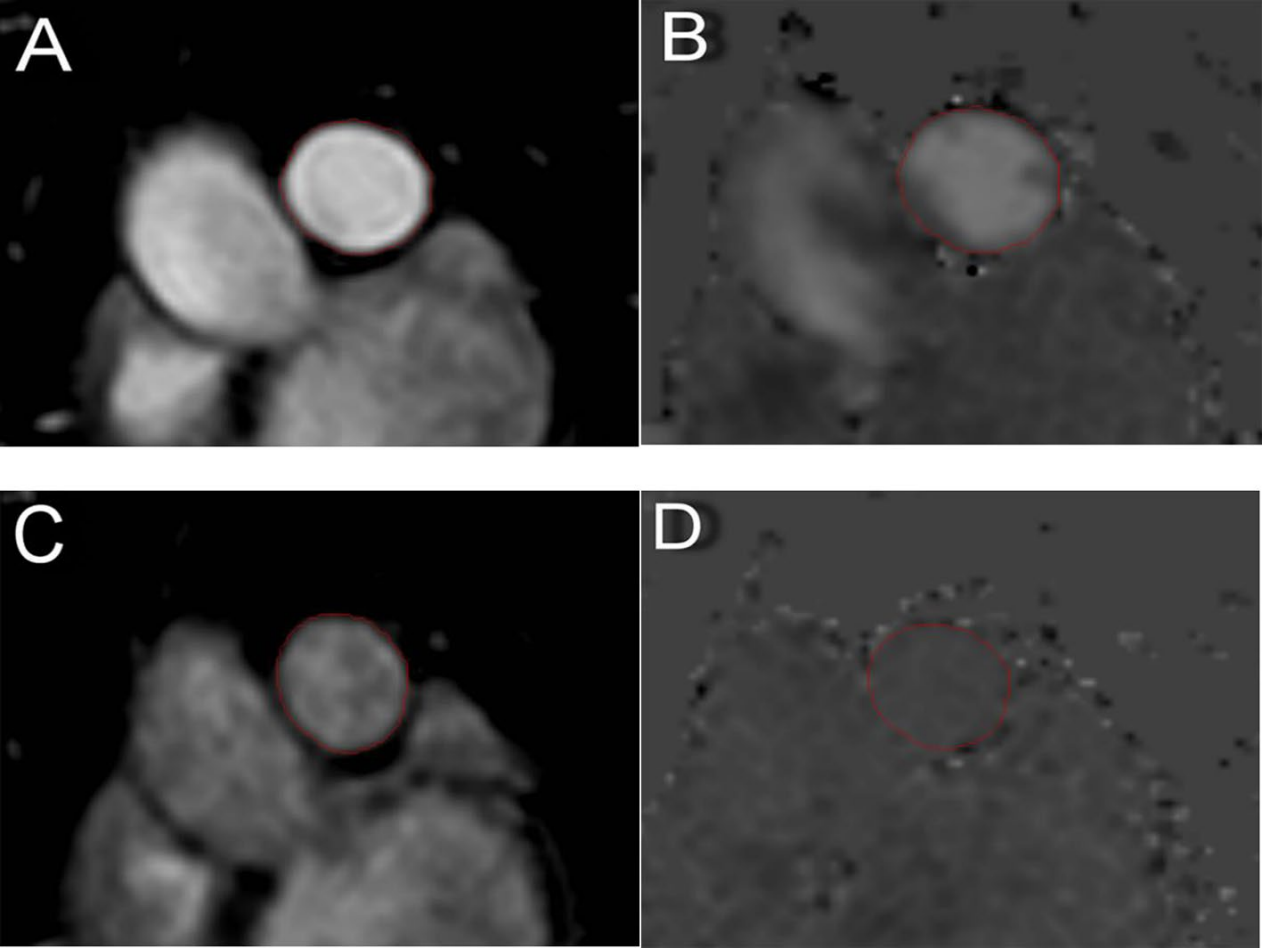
PA cross-sectional MR images. (A–D) PA cross-sectional phase-contrast MR image acquisition perpendicular to the pulmonary trunk. Cross-sectional PA areas were measured by (A) magnitude and (B) velocity images in end-systole and (C) magnitude and (D) velocity images in end-diastole. Vessel contours (red circle) were traced throughout the entire cardiac cycle. *PA* pulmonary artery.
